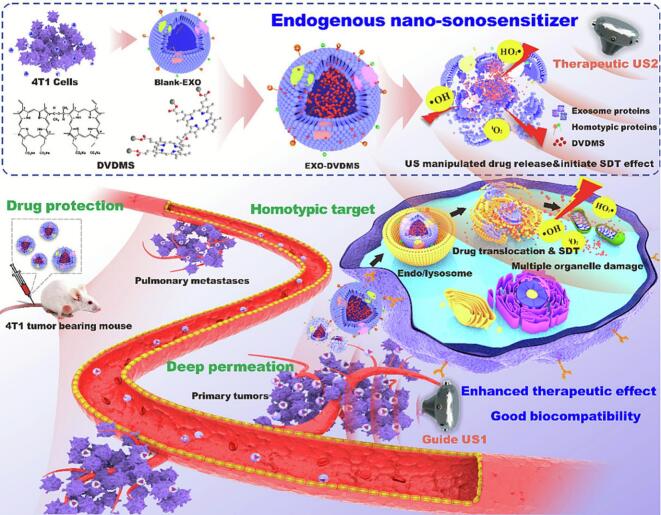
Ultrasound-triggered EXO-DVDMS selectively targets tumors, disrupts lysosomes, and releases DVDMS into the cytoplasm. The DVDMS boosts the production of reactive oxygen species (ROS) and enhances sonodynamic therapy (SDT) efficacy. Reprinted from (Liu et al., 2019); licensed under CC BY 4.0.
